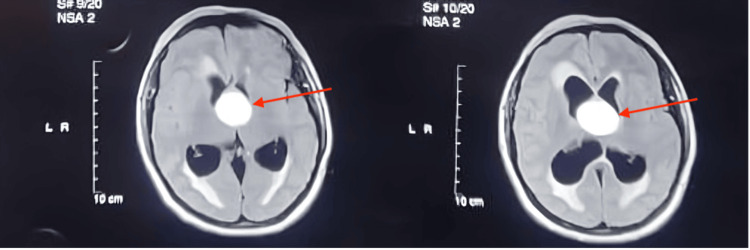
MRI images showing axial T2 FLAIR sections of the brain There is a well-defined, hyperintense cystic lesion (red arrow) located in the anterior superior portion of the third ventricle, measuring approximately 3x3 cm with hydrocephalus FLAIR - fluid-attenuated inversion recovery
